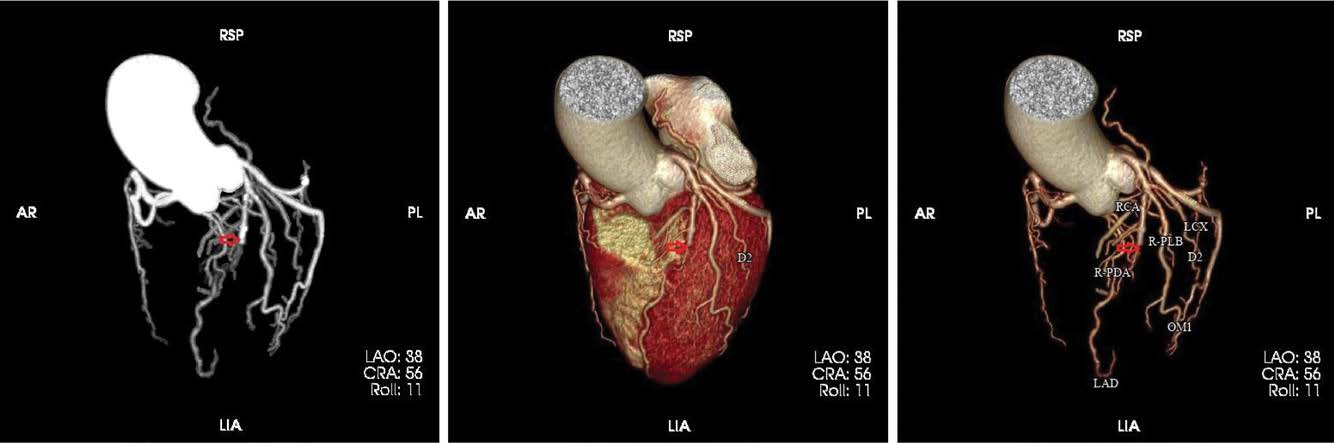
Coronary CTA. The red arrow points to an occlusion of the middle portion of the anterior descending coronary artery. CTA = computed tomography angiography.
